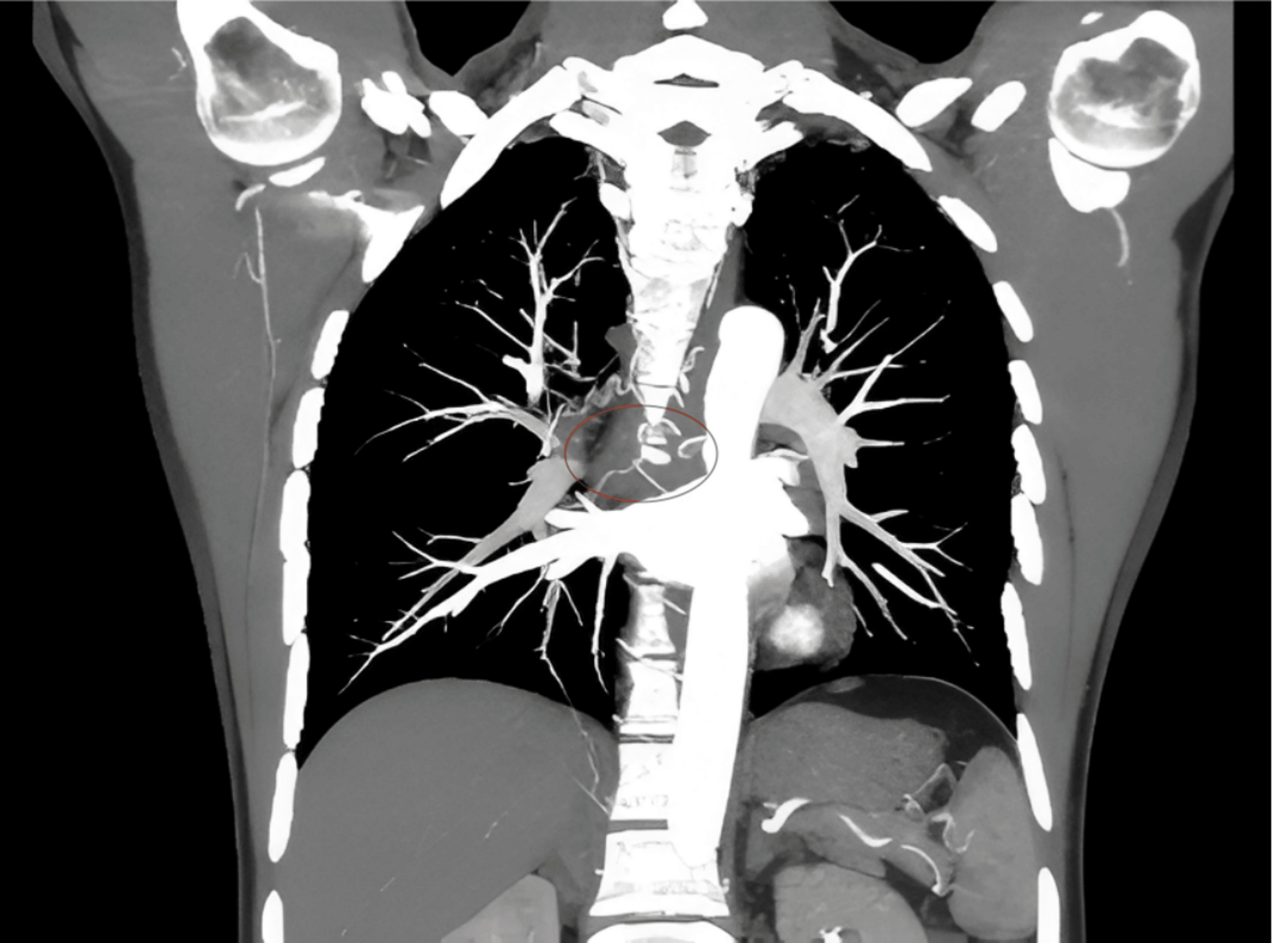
CT scan of the chest showing active contrast extravasation from the mid-thoracic esophagus (encircled), indicating ongoing active bleeding. The pulmonary arteries are opacified with contrast. There is an area of contrast extravasation near the pulmonary vessels, meaning that contrast is seen outside the normal vessel lumen, which is abnormal and consistent with active bleeding.
